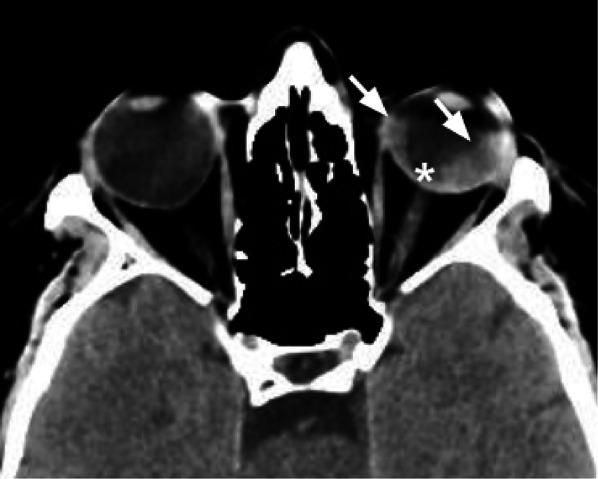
Axial CT acquisition reconstructed with soft tissues algorithm showing a left retinal detachment. Evidence of folded membranes with hyperdense fluid in the subretinal space (white arrows). The detachment converges posteriorly on the optic disc (asterisk)
